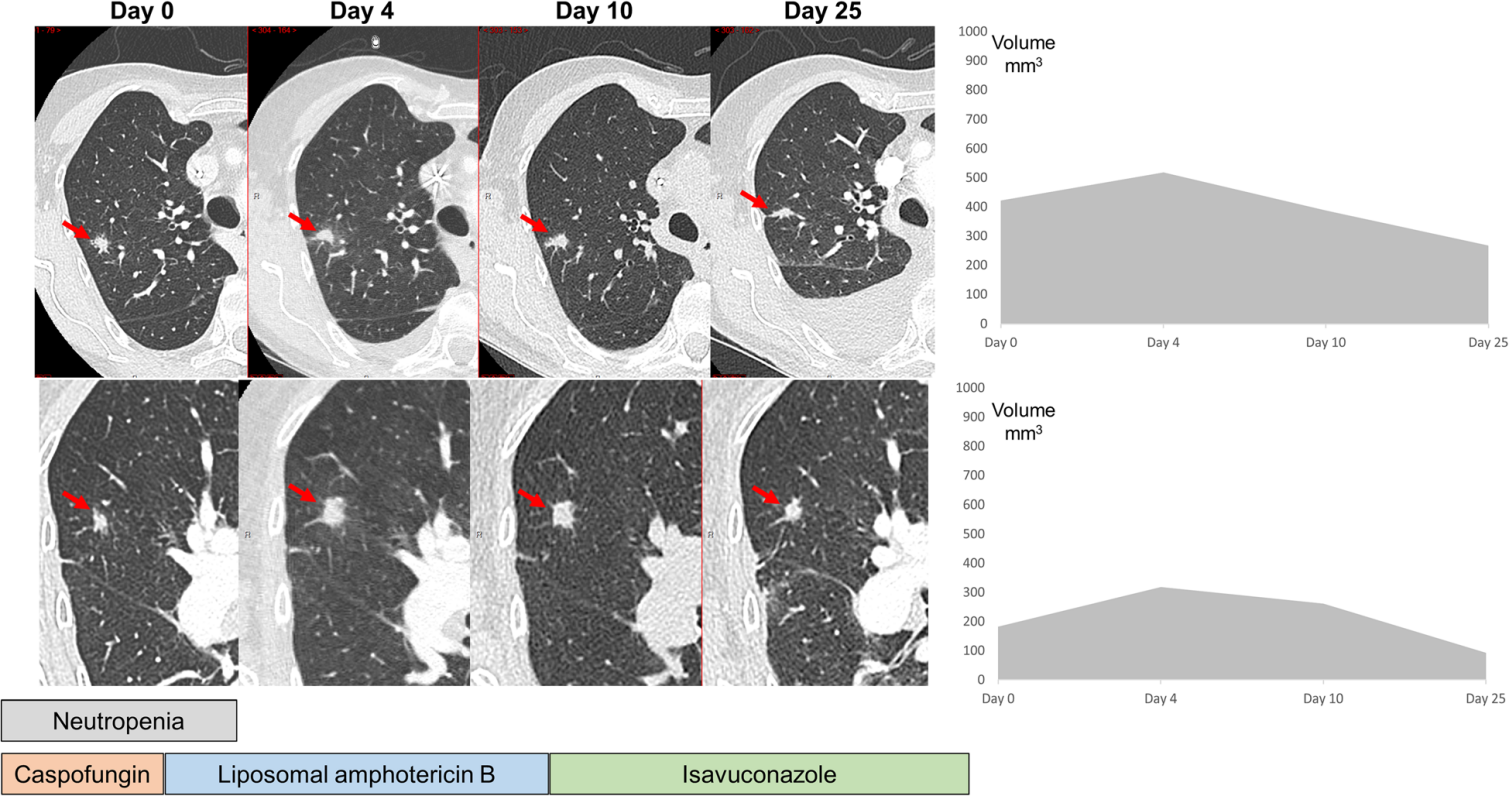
Chest CT imaging at different time points (day 0 = initial diagnosis) showing two nodular lesions in the upper right lobe (red arrows). The graphs in the right panel show volumetric data of the two lesions (volume in mm^3^) at the different time points. Duration of neutropenia (neutrophil count < 500/mm^3^) and sequence of antifungal therapy courses are shown below
